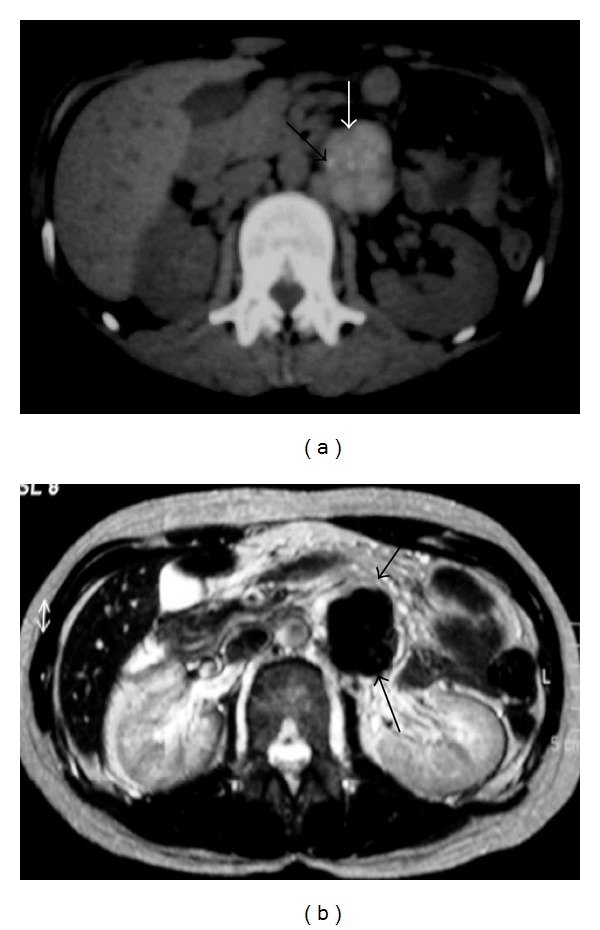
NHL. (a) Nonenhanced axial abdominal CT shows left paraaortic node (arrows) with small calcifications. (b) Axial T2-weighted image demonstrates left paraaortic hypointense mass (arrows).
